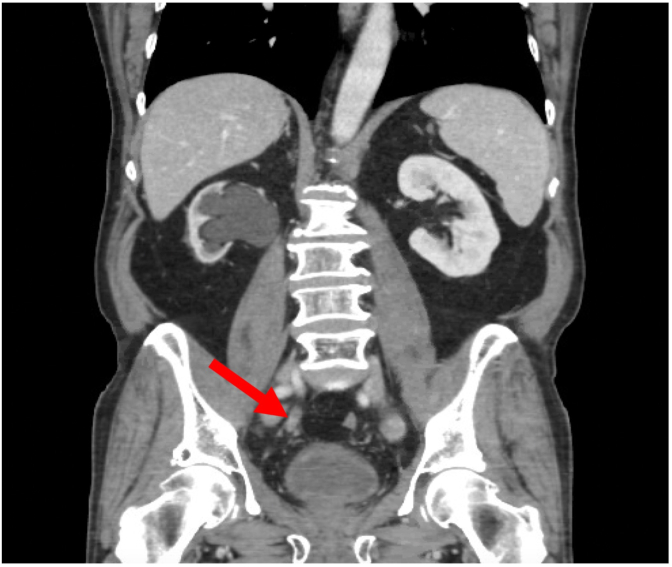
CT abdomen/pelvis revealing marked right hydronephrosis secondary to an endoluminal lesion in the distal ureter.
